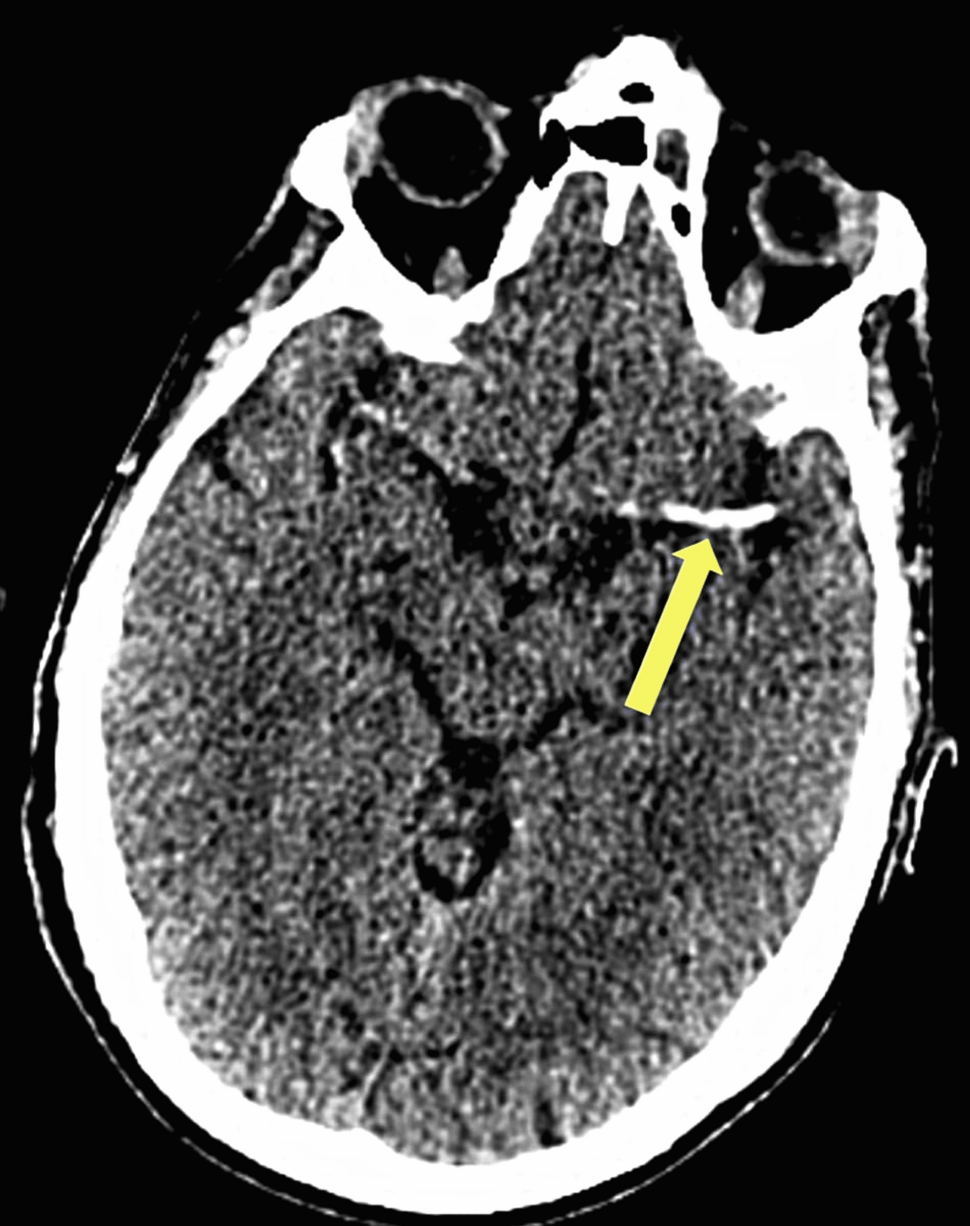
CT brain showing hyperdense left MCA CT: computed tomography, MCA: middle cerebral artery
